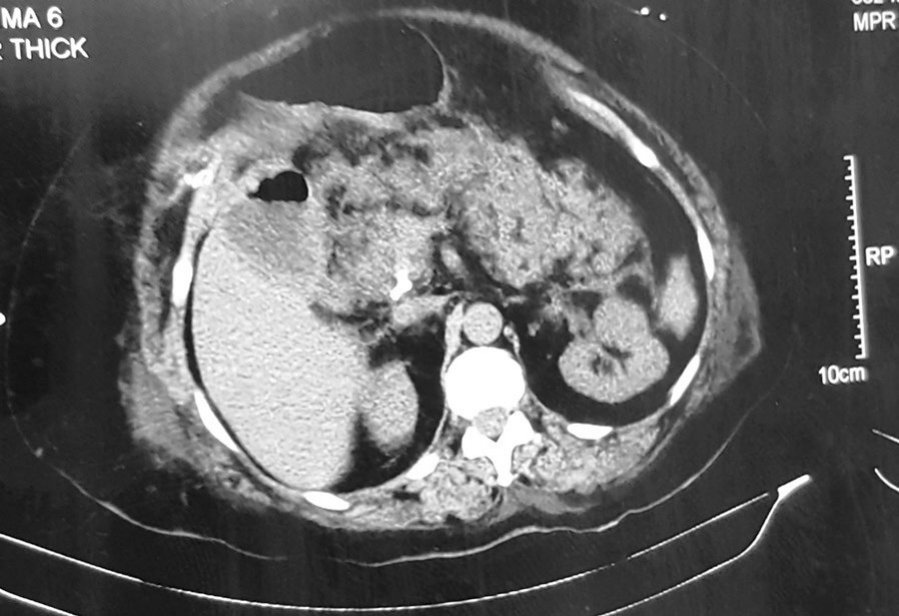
CT abdomen showing necrotizing panceatitis
